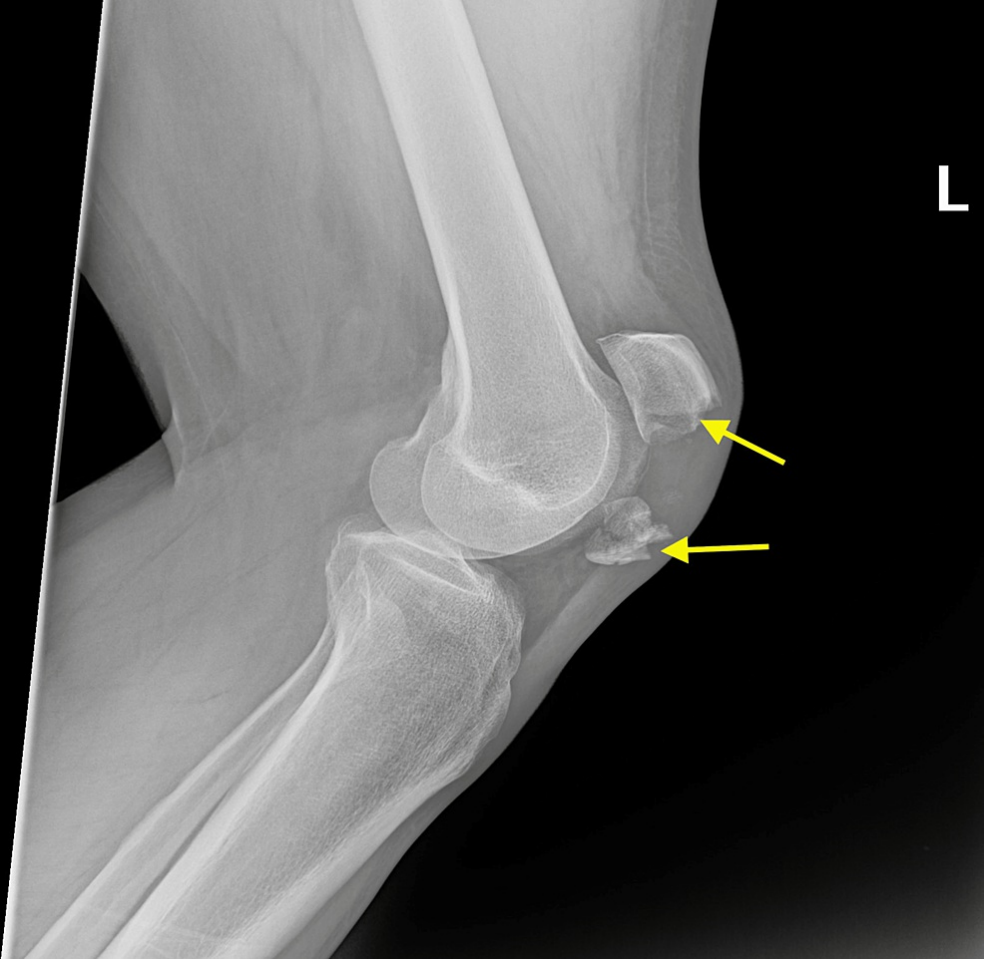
Radiograph demonstrating comminuted patella fracture
